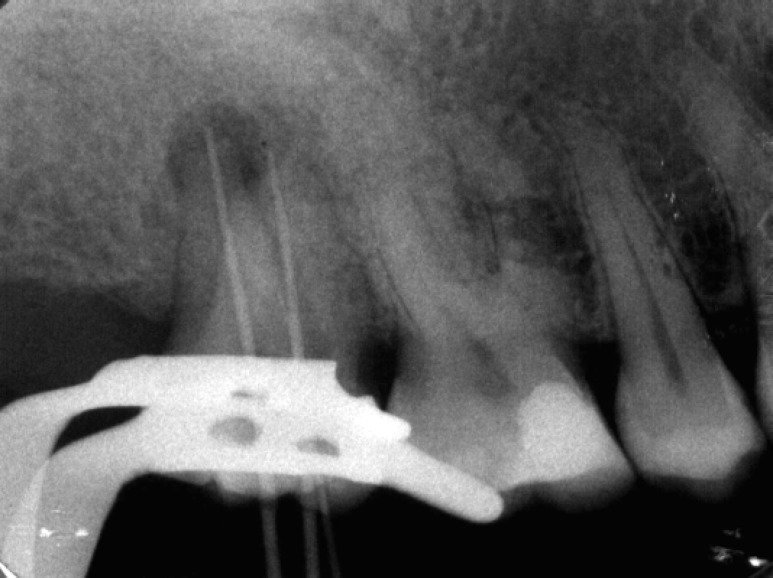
Master cone confirmation X-ray
